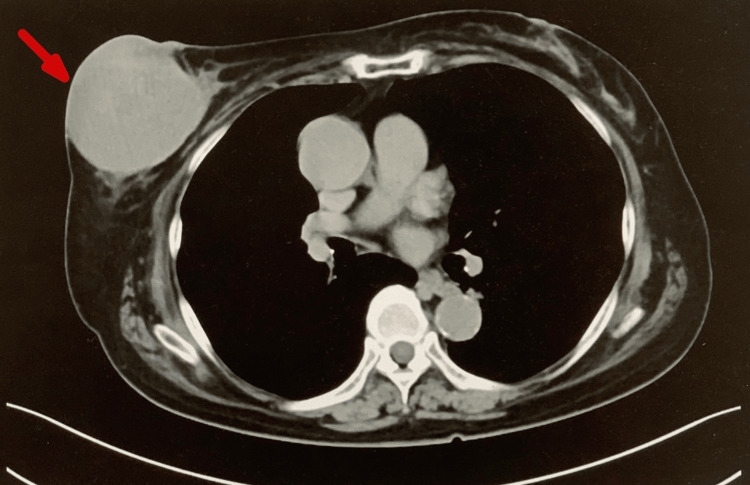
Preoperative computed tomography of the patient
